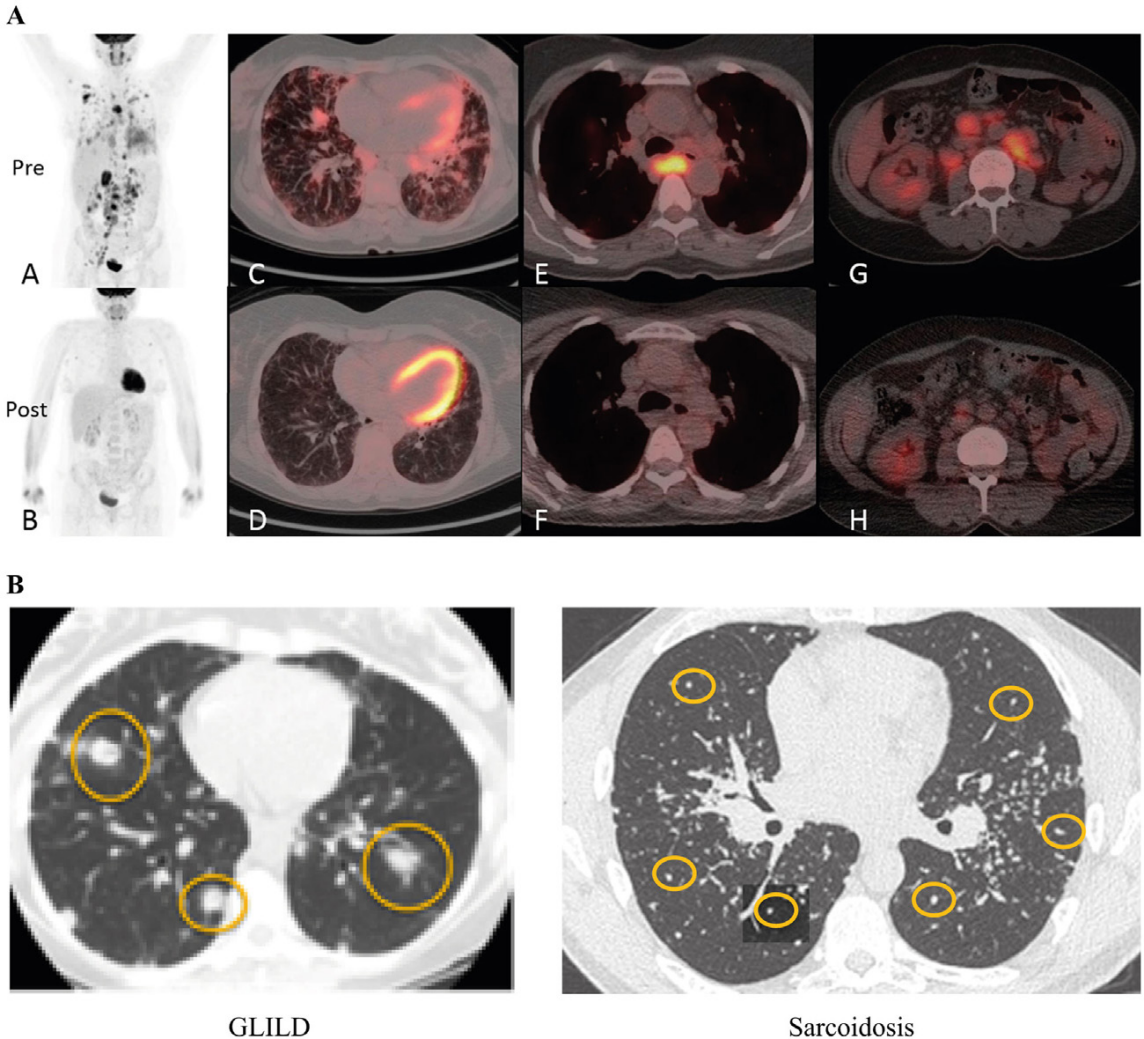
Diagnosis of granulomatous-lymphocytic interstitial lung disease (GLILD). (A) PET-CT before and 3 months after rituximab and mycophenolate treatment. Whole body images were acquired at 90 min following 285 MBq 2-[(18)F]-fluoro-2-deoxy-d-glucose (tracer). Maximum intensity projection whole body images before treatment show widespread abnormal uptake of tracer in the lung parenchyma and lymph nodes above and below the diaphragm (A); images following treatment show near resolution of all the areas of abnormal tracer uptake (B). (C,D) Axial fused positron emission tomography–computed tomography (PET–CT) images at the mid-thoracic level. The level of tracer uptake is represented by the intensity of color superimposed upon the CT image. There is a combination of interstitial septal thickening and ill-defined tracer-avid peri-bronchovascular nodules before treatment (C); post-treatment image shows improvement of the nodularity with near resolution of tracer uptake (D). Images at the level of the carina show an enlarged lymph node exhibiting intense abnormal tracer uptake prior to treatment (E), and a normalized lymph node with no abnormal tracer uptake following treatment (F). Pre-treatment images through the abdomen at the level of the right renal hilum demonstrate numerous enlarged tracer-avid retroperitoneal lymph nodes (G) which reduce in size and tracer uptake following treatment (H). Reproduced with kind permission from 2016 British Society for Immunology, Clinical and Experimental Immunology, 187: 138–145 (100). (B) Radiographic features of GLILD versus sarcoidosis. GLILD is characterized by macronodular (yellow circles) disease that is located predominantly in the lower lung zones. In contrast, micronodular (yellow circles) disease located in the upper lung zones and frequently accompanied by marked hilar adenopathy (stars) is characteristic of sarcoidosis. Reproduced from Ref. (101) with kind permission from European Respiratory Society.
